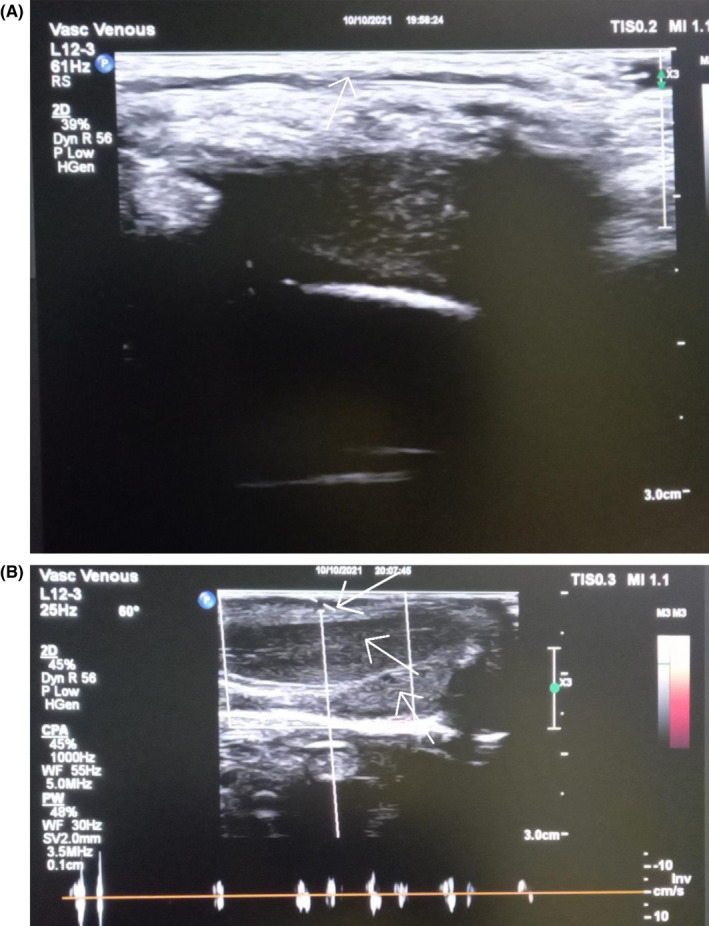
Demonstrating sonographic and Doppler images. (A) The white arrow pointing to the dilated superficial penile dorsal vein. (B) The upper white arrow pointing to the dilated superficial penile dorsal vein with evidence of venous flow and no thrombus; the middle arrow to the corpus cavernosum and the lower arrow to the corpus spongiosum
